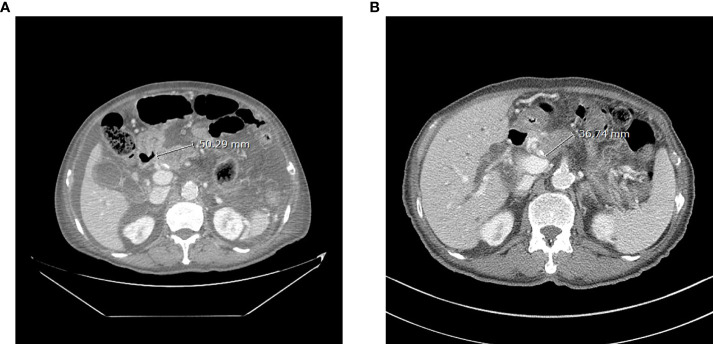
Abdominal CT of the patient before (A, 7 July 2021) and after (B, 6 September 2021) 8 weeks of dabrafenib use.
